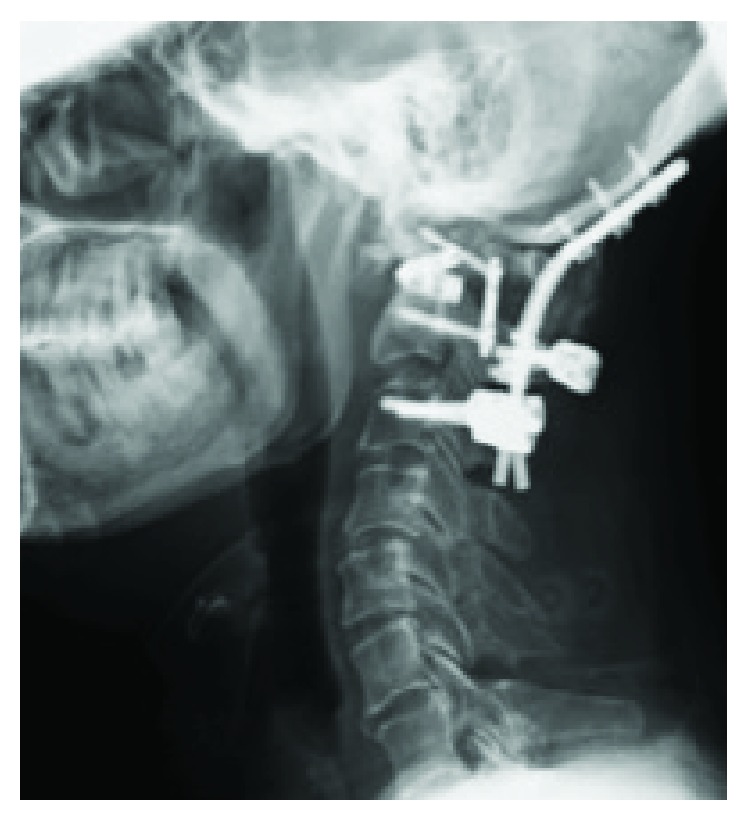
Lateral cervical X-ray after the posterior O-C3 fusion surgery at our hospital showing O-C2 angle of 6-degree lordosis.
